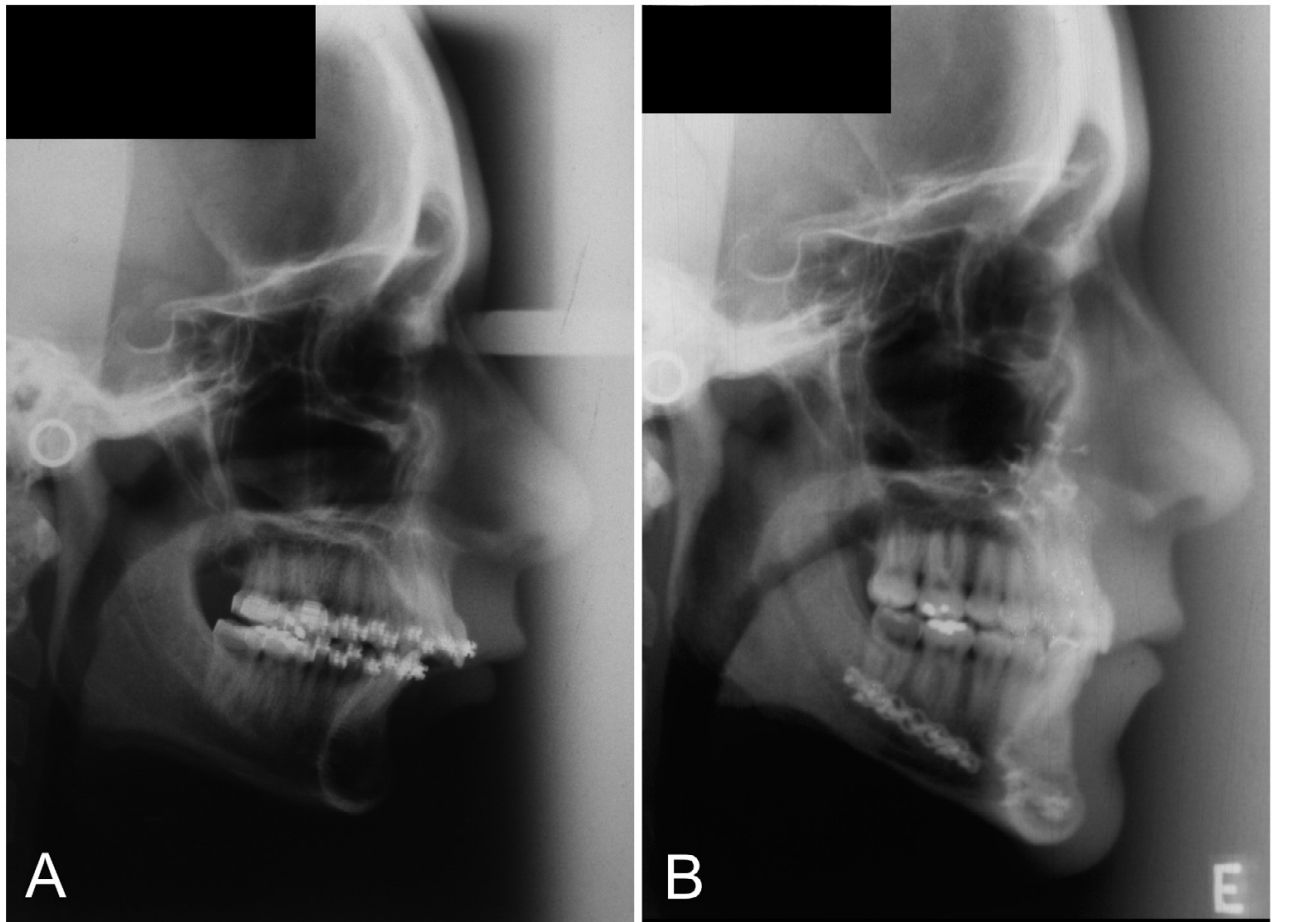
Radiographic comparison. A. Lateral extra-oral radiograph for preoperative cephalometric investigation of a retrognathic patient. Skeletal image of the retropositioned mandible can be seen in type II occlusal relationship. Soft structures show characteristic deep mentolabial sulcus and small facial height. B. Postoperative lateral extra-oral radiograph. Alveolar osteotomy can be seen from 32 to 42, associated to advancement and clockwise rotation of the mandible making up a maxilla-combined surgery. The surgery begins at the mandible. Rigid fixation miniplates measuring 2 mm are used in an extension of six holes for an advancement of 13 mm. On the maxilla, 1.5 mm rigid fixation may be observed. Soft tissue profile in accordance with skeletal results. In the naso-oro-hypopharyngeal regions, pre- and postoperative images show transversal increase of the area. This result is supported by respiratory improvement, as clinically reported by the patient.
